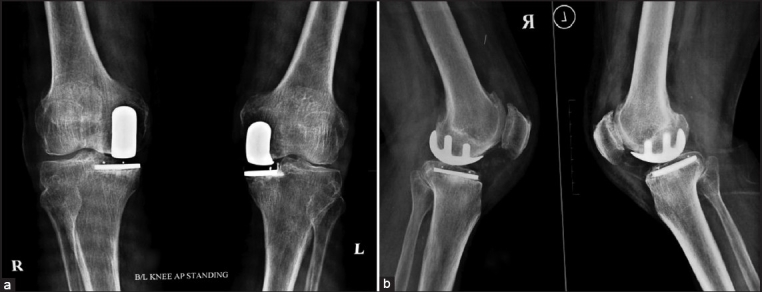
Anteroposterior (a) and lateral (b) radiographs of same patient 48 months post-bilateral UKA
